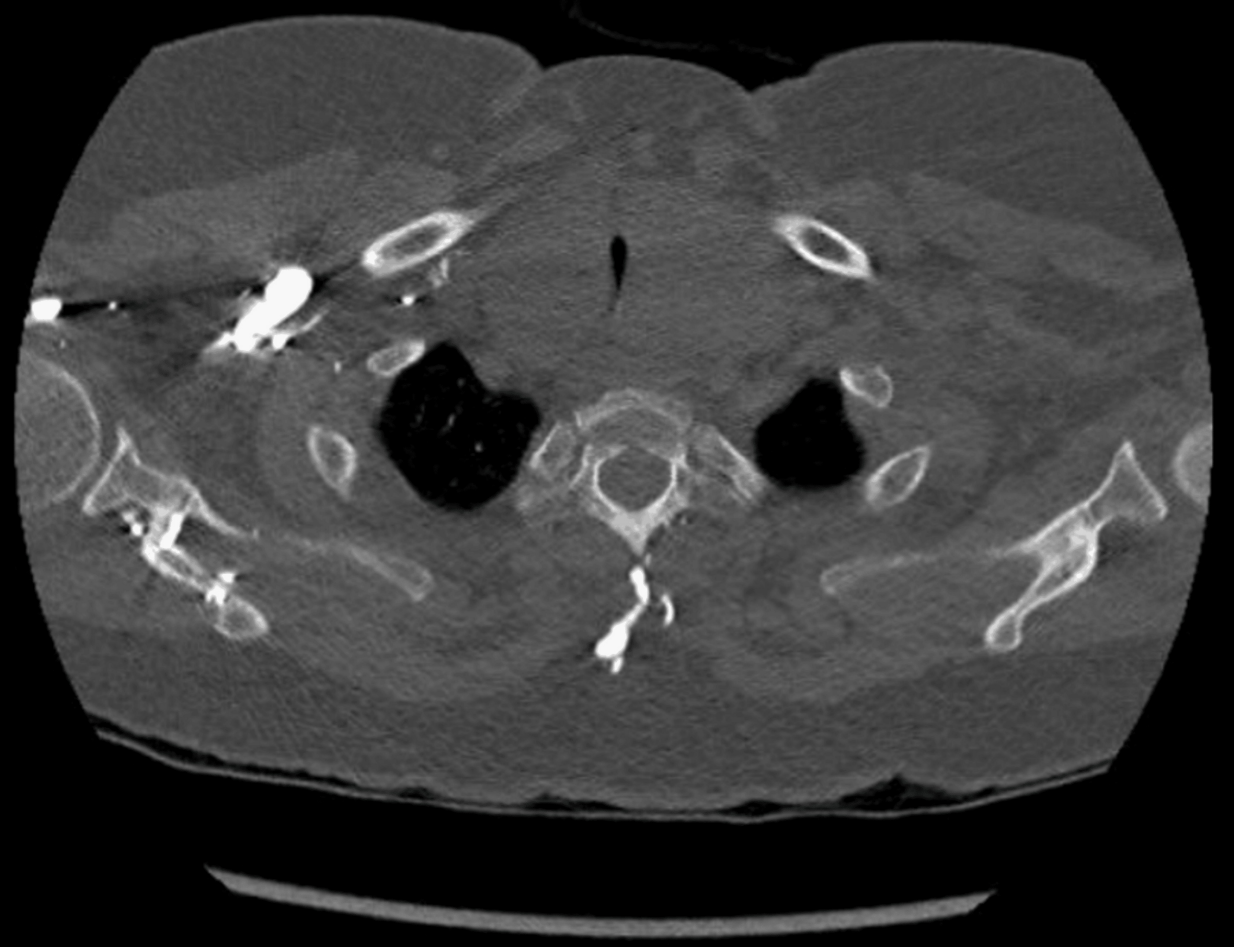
CT image showing a pronounced goiter on both sides with significant tracheal stenosis
